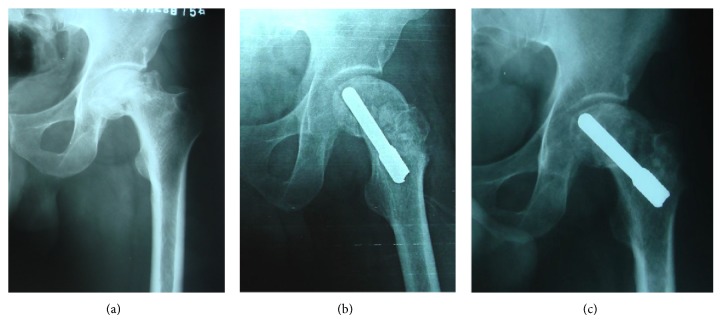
A 40-year-old male patient with ARCO stage IV ONFH at right received the treatment of BMSCs associating with porous tantalum rod implantation combined with vascularized iliac grafting. Radiographs were taken before operation (a), 18 months after operation (b), and 54 months after operation (c). (c) The femoral head remained circular, but collapse was found again in the femoral head.
